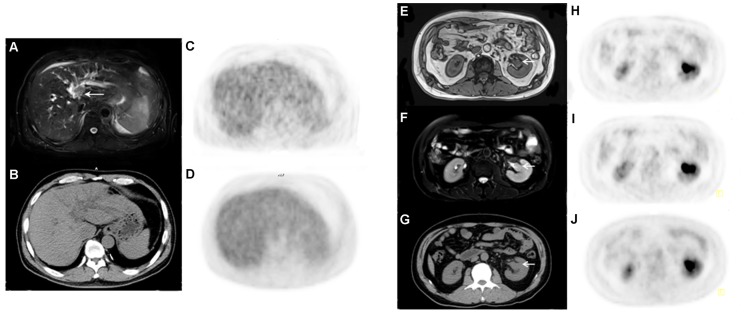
Representative cases of PET/MRI. In a 52-year-old symptom-free male, ultrasonography incidentally revealed a dilated bile duct and CT a dilated common bile duct. T2WI-FS outlined a mass inside the dilated bile duct (*arrow head*), which was later removed by surgery and the lesion was proven to be an epithelial cancer of bile duct origin (A). An right renal pelvic mass was better delineated against hot urine on T2WI-FS in a 60-yr-old woman; an invasive ureteral epithelium carcinoma was later proved by surgery (*cross,* B).
